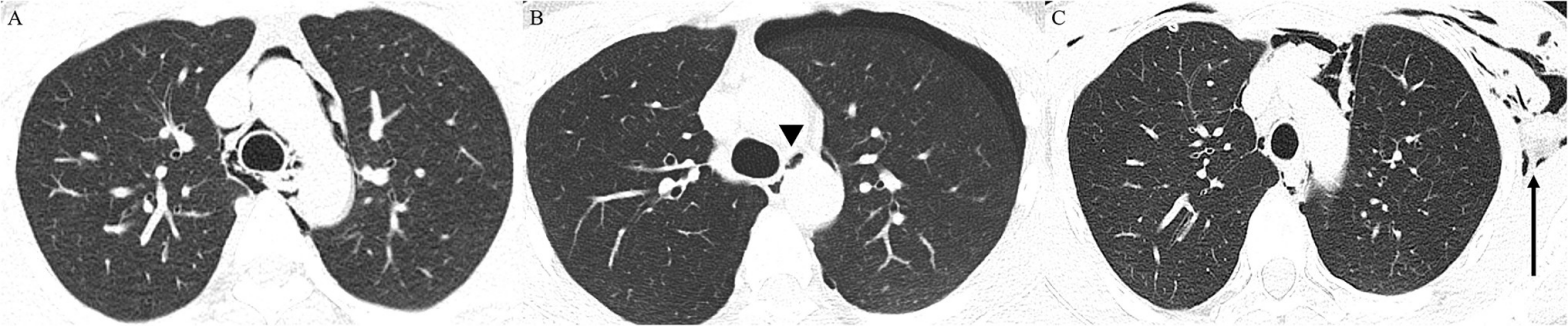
Representative cases of primary spontaneous pneumomediastinum (PSPM) on chest computed tomography (CT). (A) pneumomediastinum without other combined complication, (B) pneumomediastinum (arrowhead) with left pneumothorax, and (C) pneumomediastinum with subcutaneous emphysema (arrow) at anterior chest wall (left > right).
